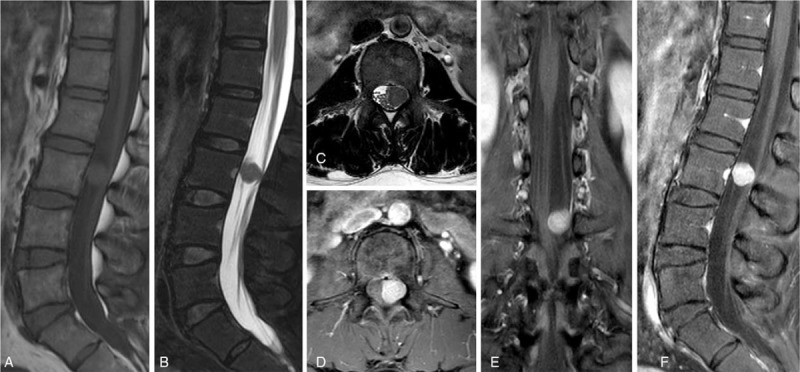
Preoperative spinal MRI showing a well-circumscribed enhancing mass at the L3 level. The mass, which was isointense on T1- and T2-weighted images (WI), homogeneously enhanced on gadolinium-contrast T1-WI, pushed the cauda equina to the right side, and occupied half the spinal canal volume. (A) Sagittal T1-WI; (B) sagittal T2-WI; (C) axial T2-WI; (D) axial contrast-enhanced image; (E) coronal contrast-enhanced image; (F) sagittal contrast-enhanced image. MRI = magnetic resonance imaging.
